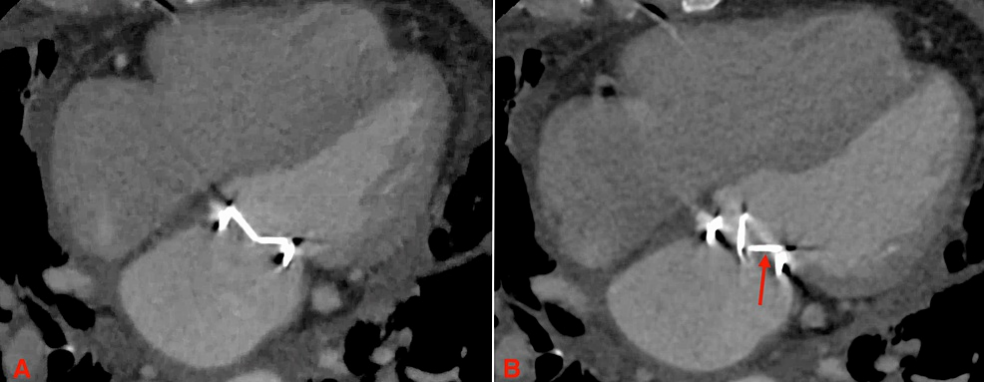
Structural computed tomography of the heart. (A) Appropriately closed mechanical mitral valve leaflets during systole and (B) fixed valve leaflet (red arrow) during diastole with narrowing of the mitral opening.
